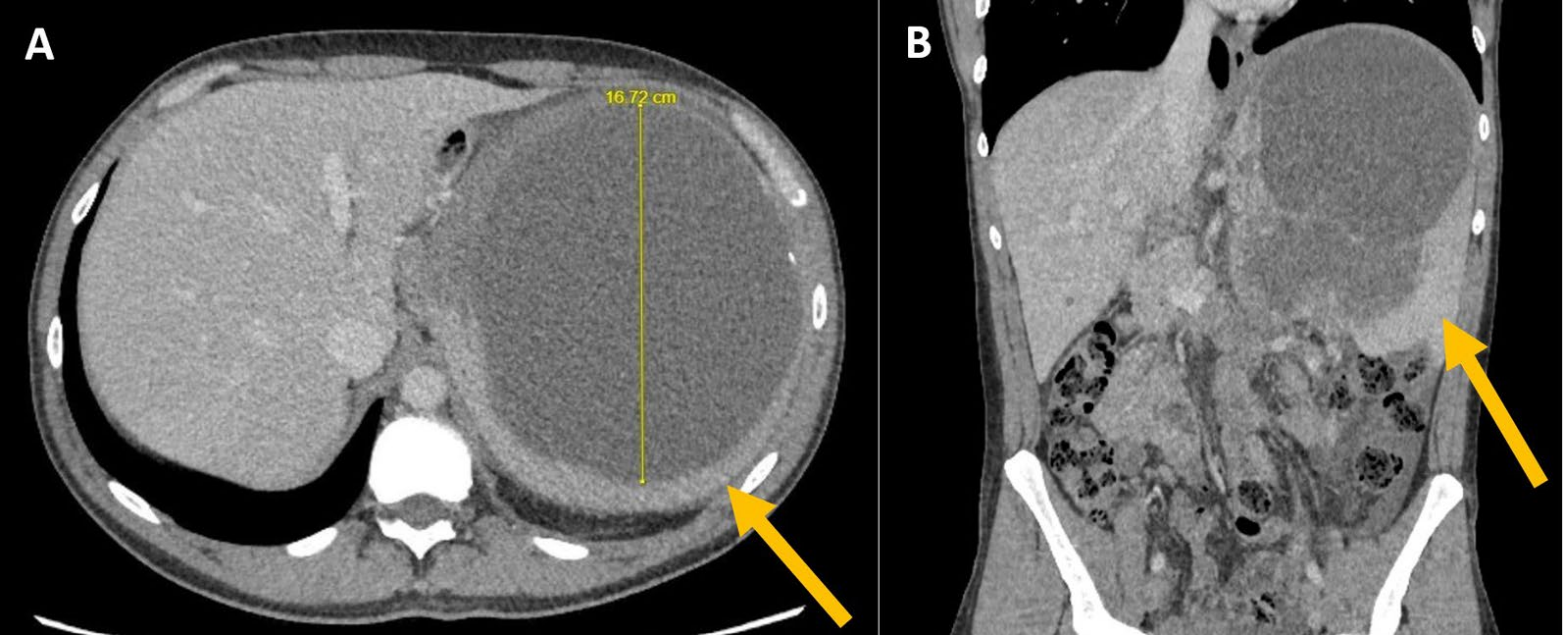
CT axial (A) and coronal view (B) demonstrating splenic size
